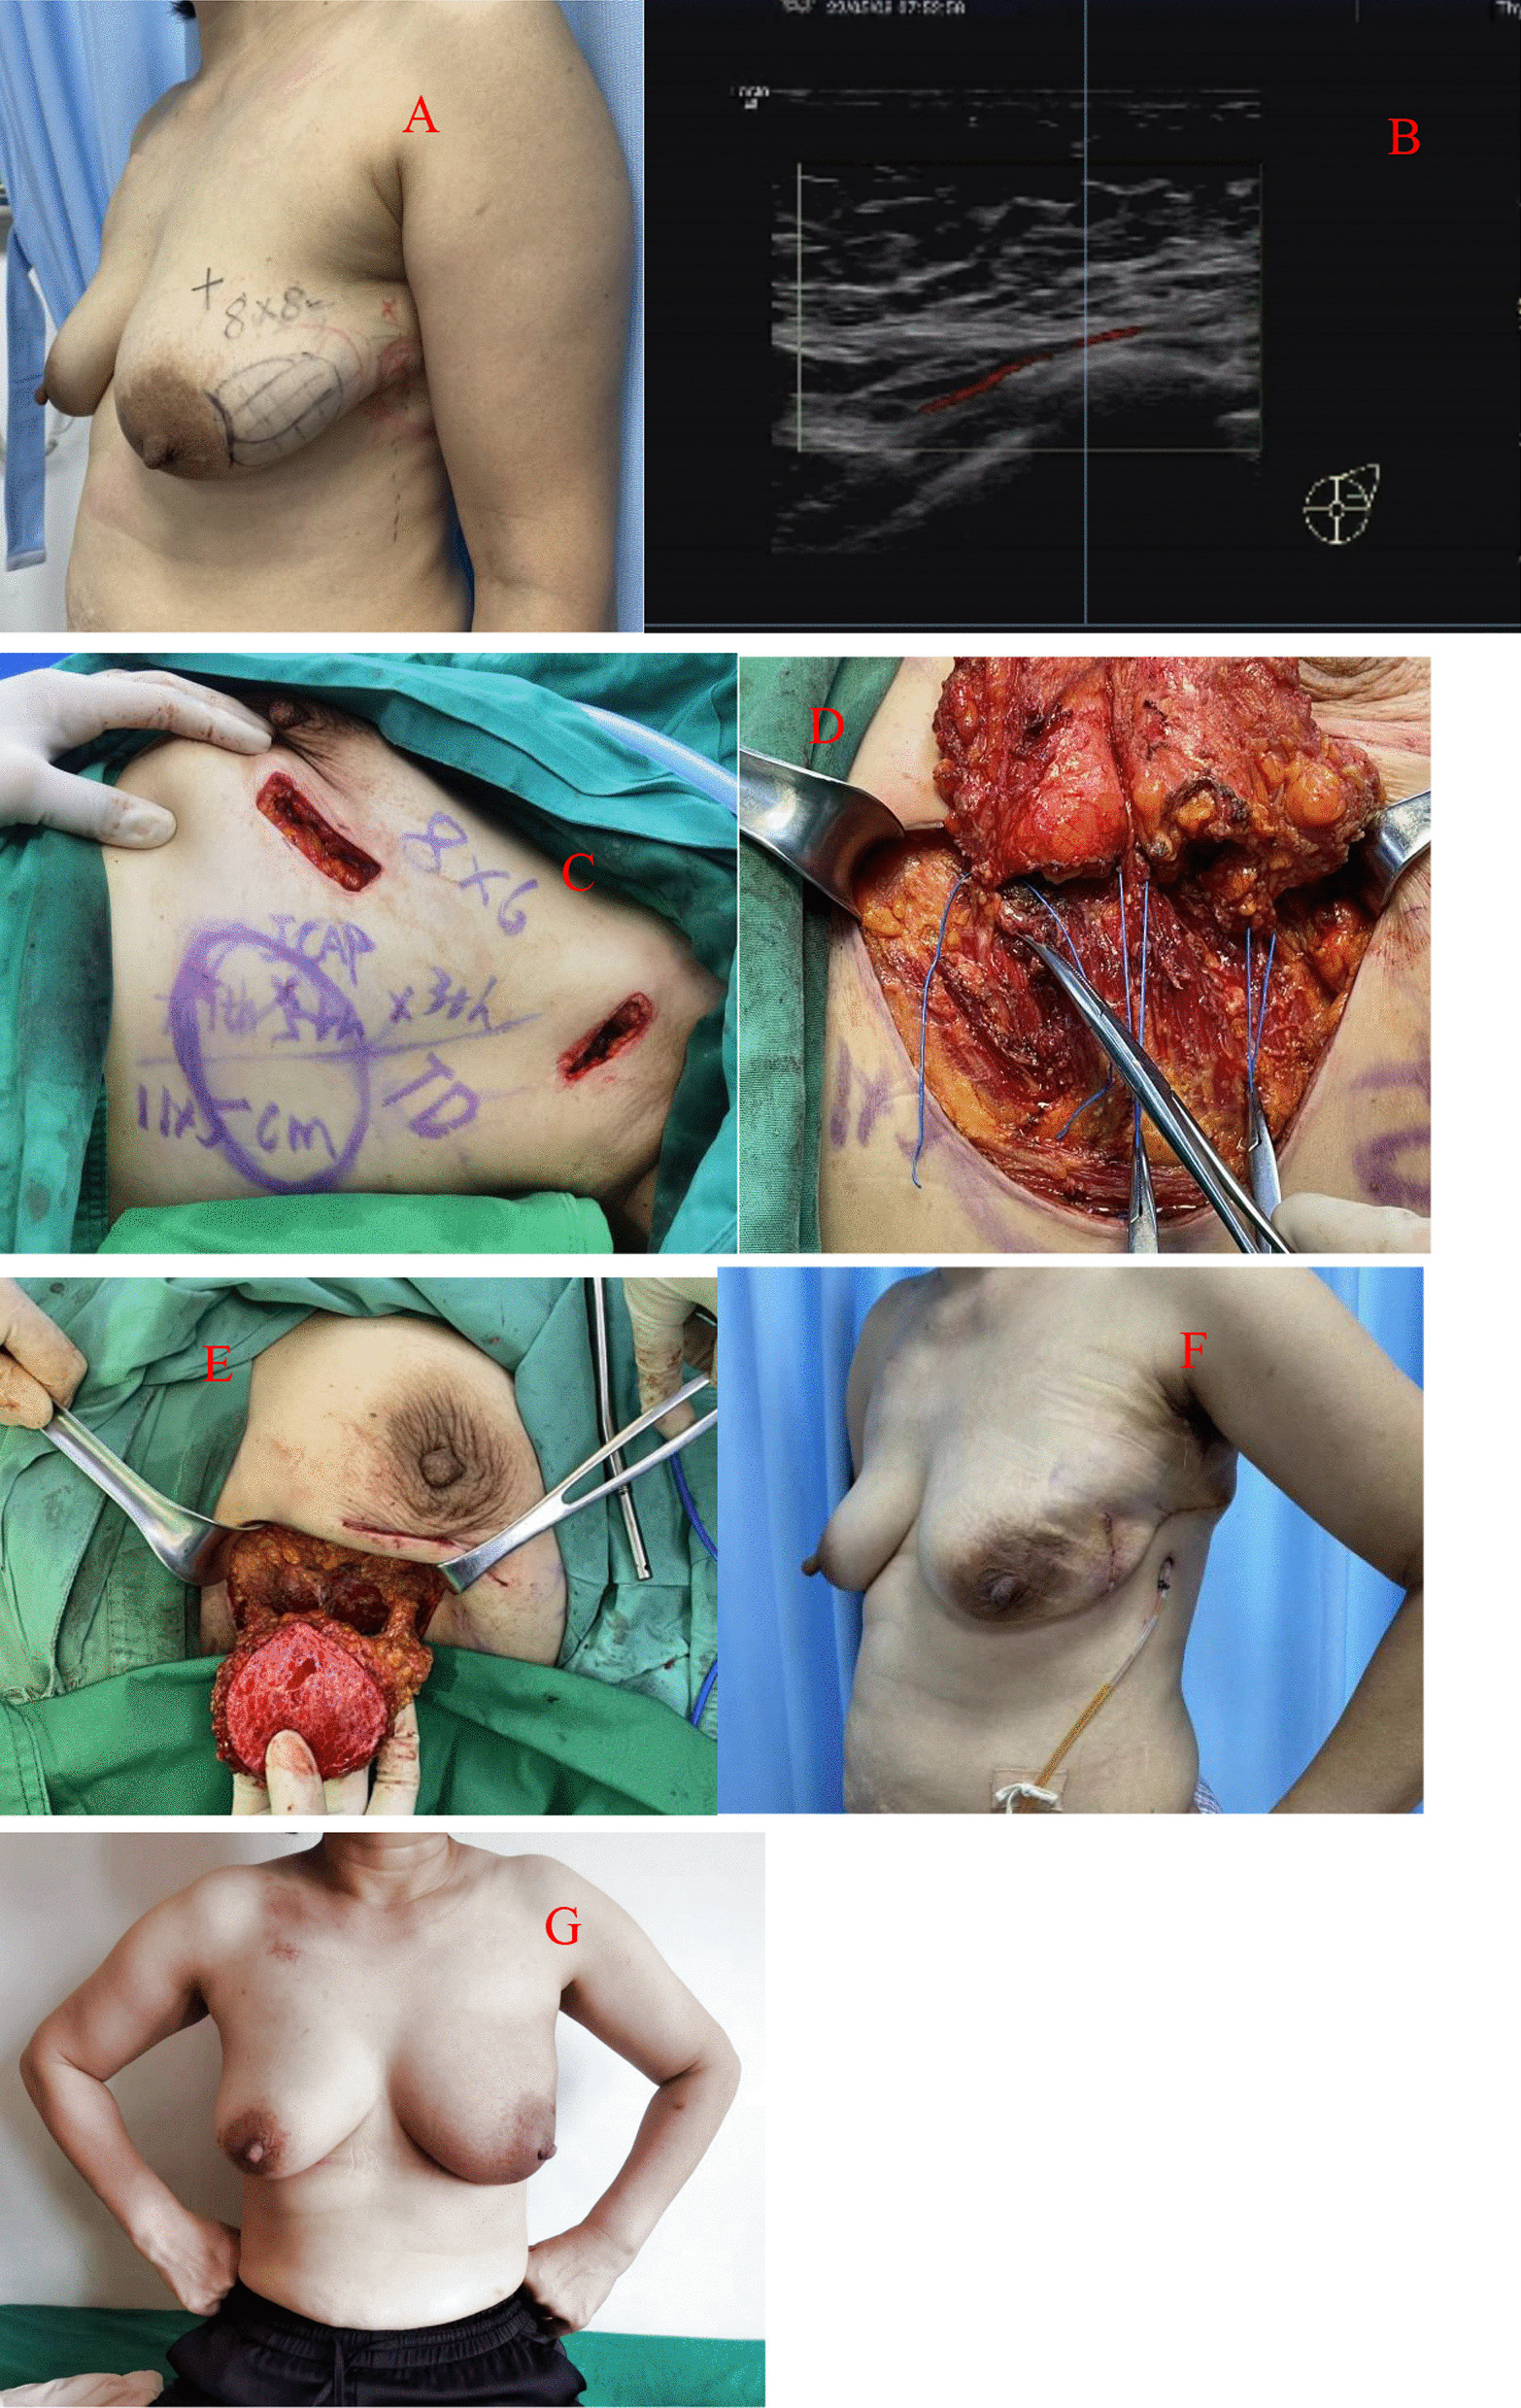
LICAP—A The precise location of the tumour was outlined. B The 5th lateral intercostal artery perforator in a colour Doppler ultrasound image. C Designed donor site to repair the defect. D The 4th, 5th, and 6th intercostal artery perforators were found intraoperatively, and a vessel belt was used to protect them months after the operation. E De-epithelialization of the flap. F Two weeks after the operation, the patient experienced fat liquefaction. G Three months after the operation
